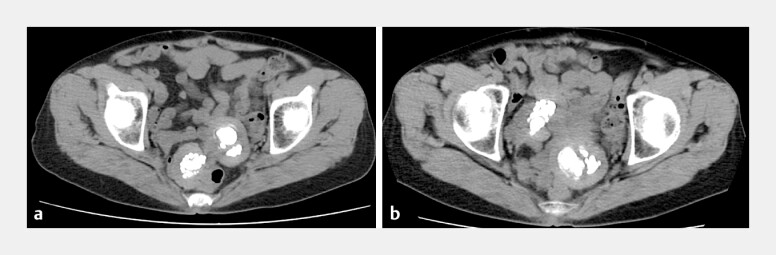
Unenhanced computed tomography (CT) images of the pelvis show calcified and mobile masses.
